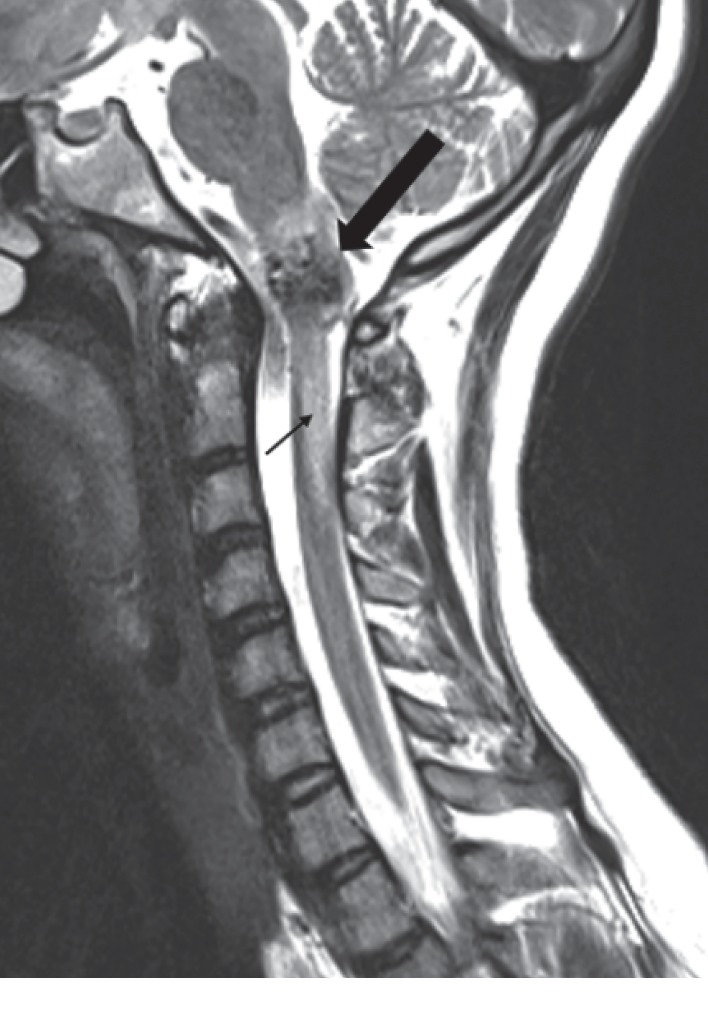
Sagittal T2-weighted MR image of the cervical spine demonstrating the expansile nidus of flow voids at the cervicomedullary junction (large arrow). Edema in the adjacent brainstem extends into the upper cervical spinal cord to the C3 level (thin arrow).
